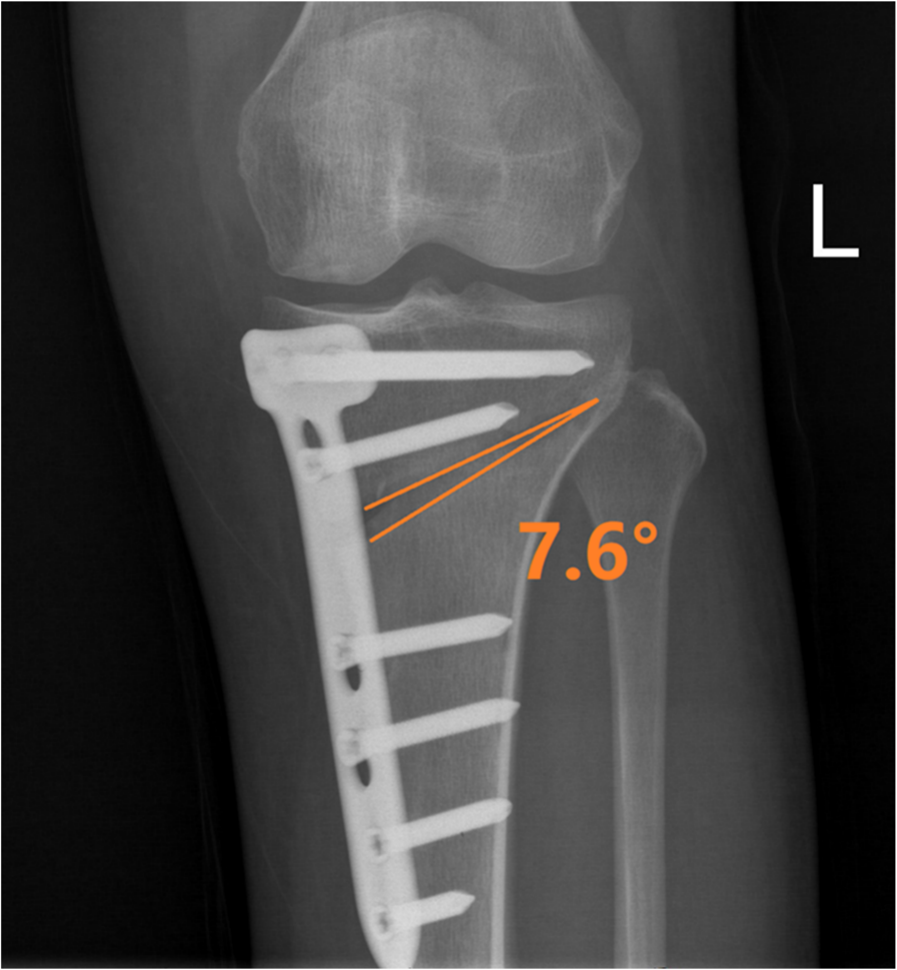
The correction angle was measured by standard orthotopic radiographs
